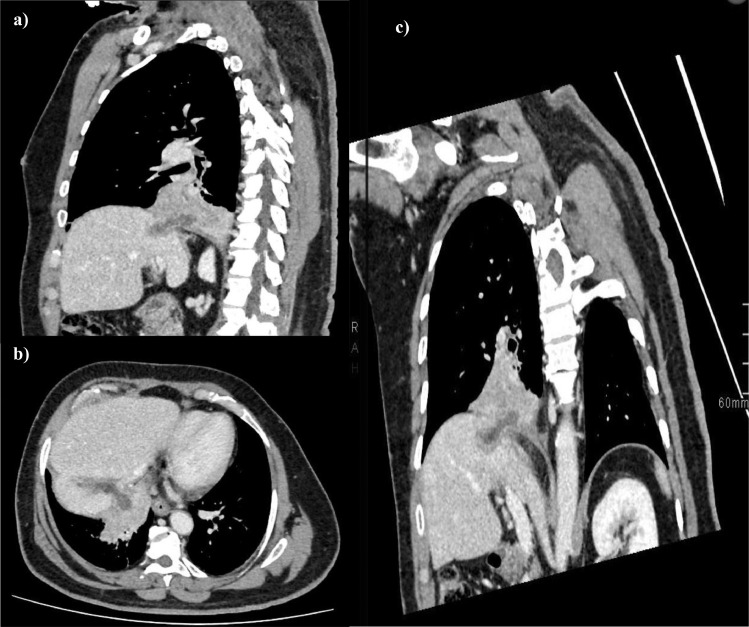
Biliopleural fistula post-RFA. Enhanced CT chest sagittal (A), axial (B), and coronal oblique reformat (C). There is fluid attenuation tract extends from the liver dome to the right lower pleural cavity with secondary right lower lobe mass-like consolidation.
